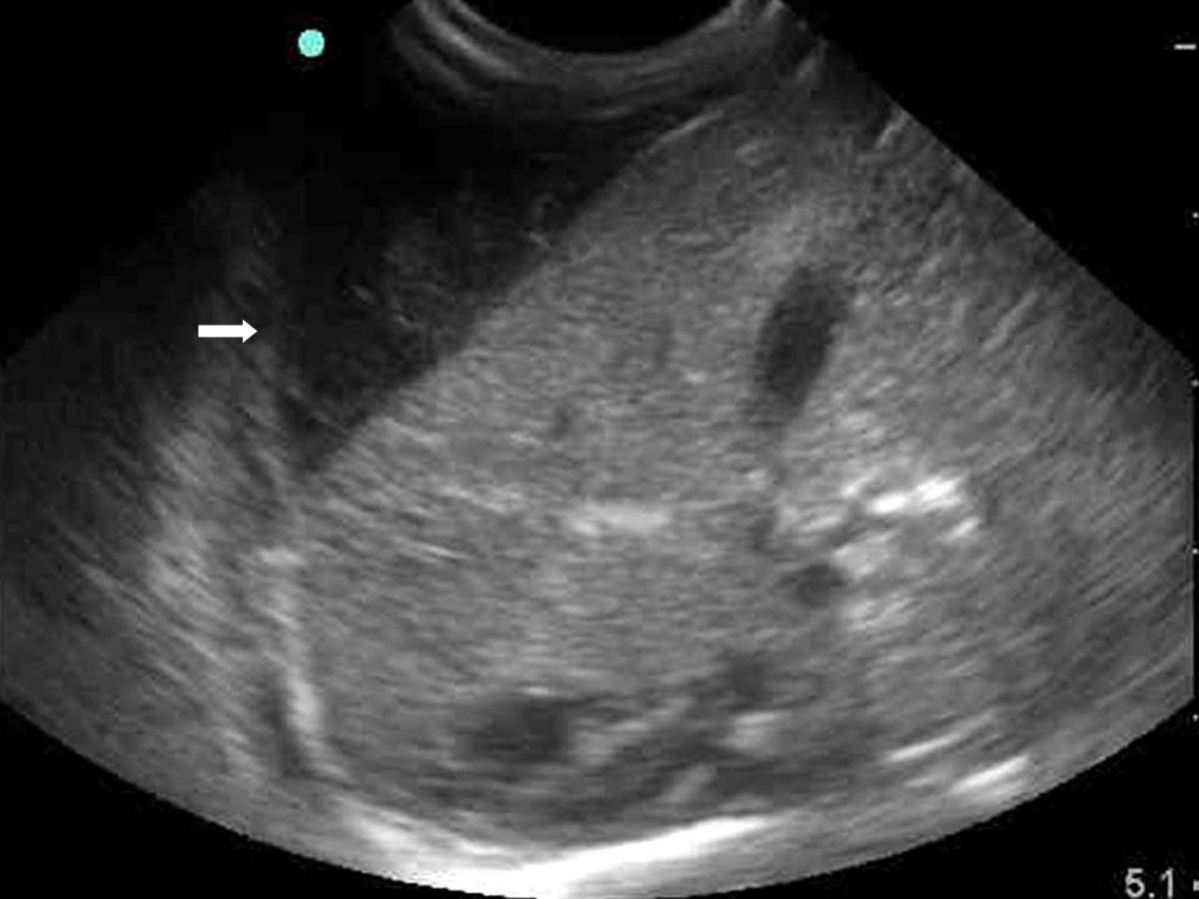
Imaging findings are shown for a subcapsular hematoma in a one-day old infant. An abdominal sonogram shows a cystic mass containing an internal thin septum with compression of the liver parenchyma in the right lobe of liver (arrow).
